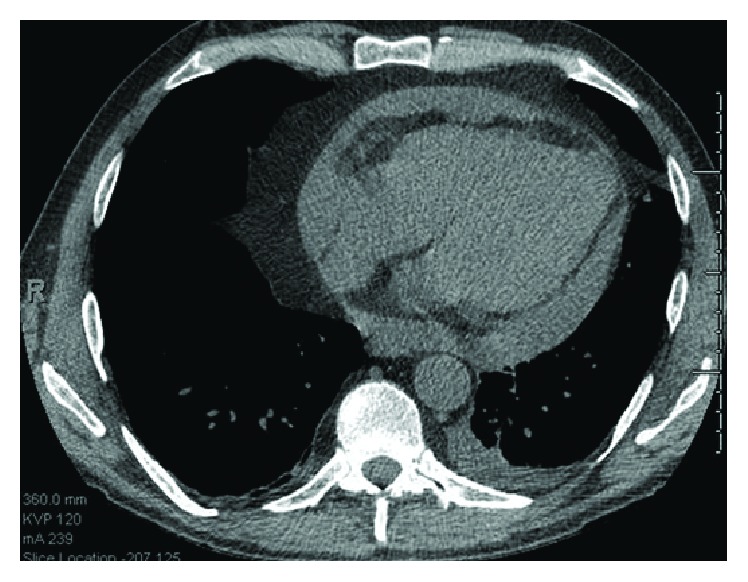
CT of the chest demonstrates a new, moderate, loculated, pericardial effusion and a small left-sided pleural effusion. Right and left atria are normal in diameter.
